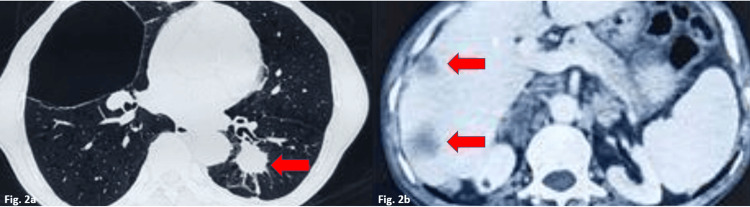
2a-Contrast tomography (CT) images of the chest showing ill-defined heterogeneous enhancing hypo-dense lesions with speculated margins in the left lower lobe measuring 26x18 mm, likely of primary malignant etiology. 2b - CT abdomen showing multiple hypodense heterogeneously enhancing lesions in both lobes of the liver and bilateral, bulky, moderately enhancing adrenal glands, most likely metastasis.
